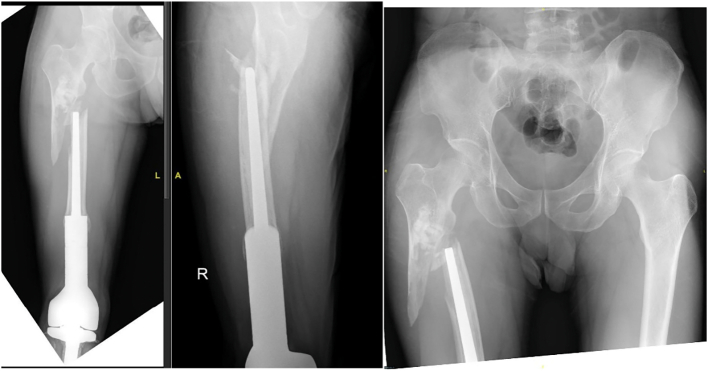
Plain radiograph showing periprosthetic fracture at the right proximal femur.
